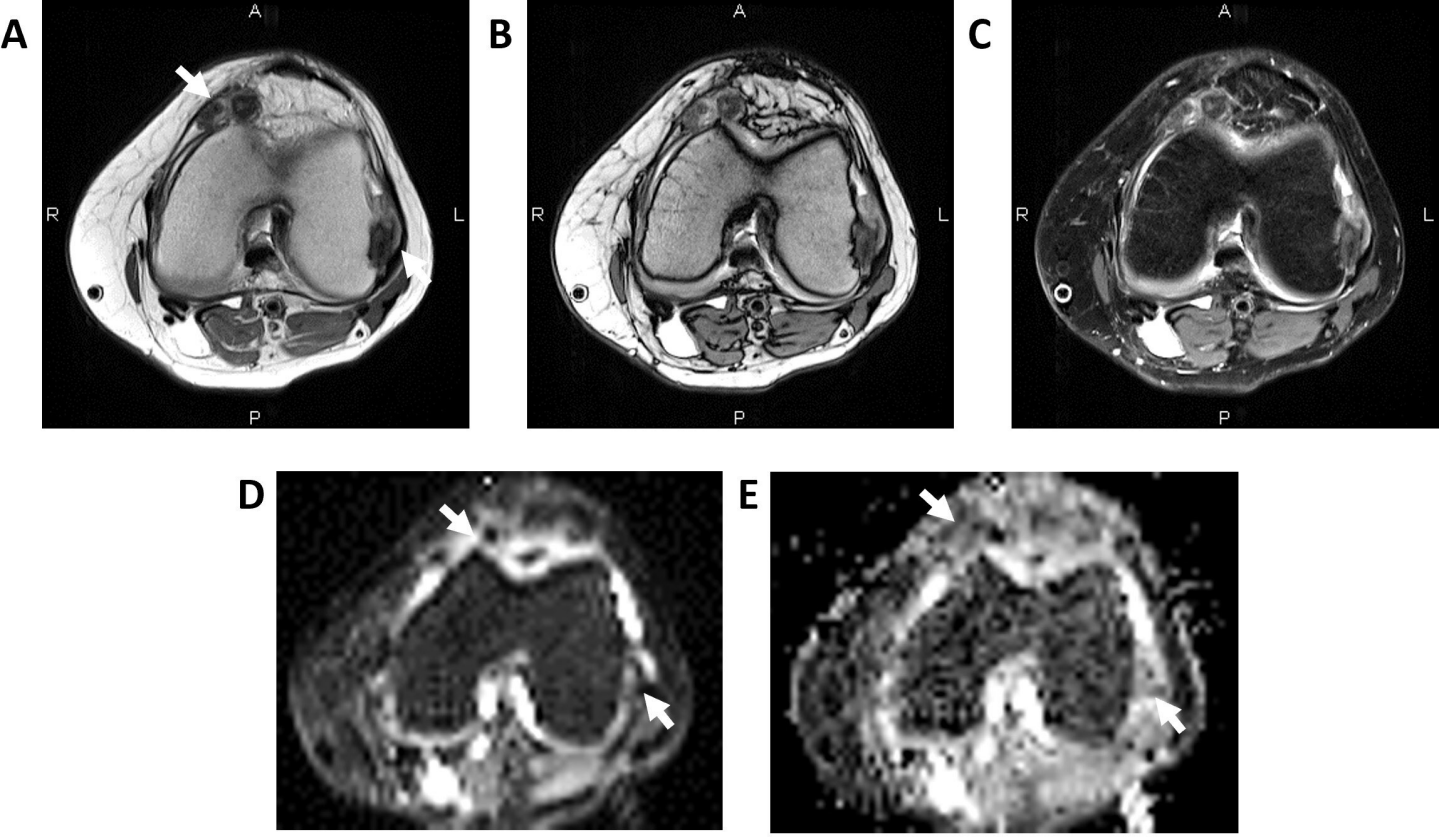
Tenosynovial giant cell tumor/PVNS (A–C). Axial T2-Dixon images (in-, opposed-, and water-maps) through the knee show mixed hypointense intra-articular lesions (arrows) suggesting PVNS. Corresponding DWI (D) and ADC (E) images show dark SI on both images (T2-black through effect) consistent with multifocal/diffuse PNVS (arrows). ADC, apparent diffusion coefficient; DWI, diffusion-weighted imaging; PVNS, pigmented villonodular synovitis.
